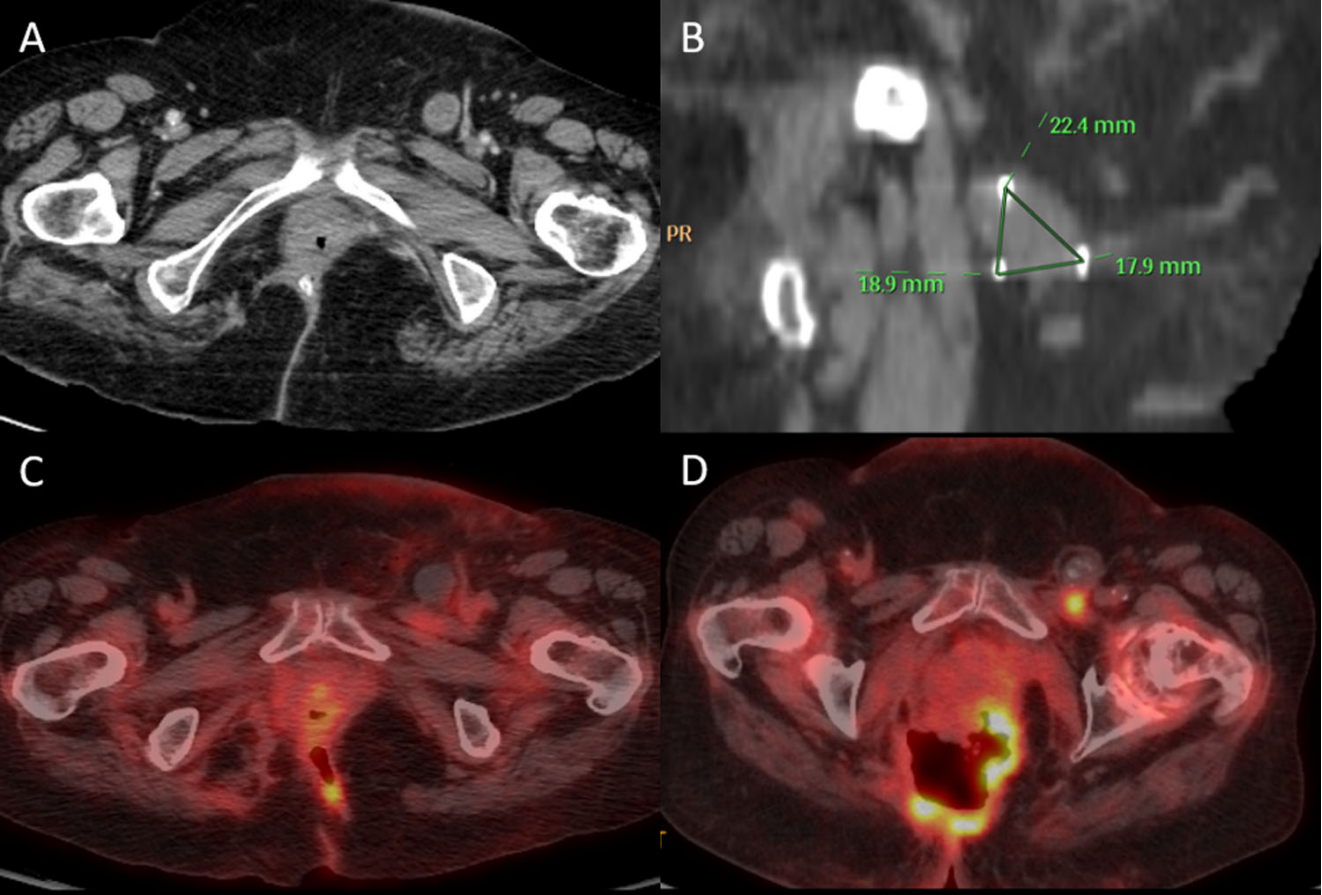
A 66-year-old female admitted with rectal pain. Past medical history of adenocarcinoma of the rectum diagnosed in 2019, underwent loop sigmoid colostomy, completed radiation and is receiving adjuvant chemotherapy (FOLFOX) upon treatment. (A) Abdominopelvic CT shows a left inguinal lymph node measuring up to 2.7 cm concerning for metastasis. Ultrasound-guided biopsy of the left inguinal lymph node with frozen samples was positive for adenocarcinoma. (B) Intraprocedural CT demonstrating intermittent placement of three 17-gauge NanoKnife IRE ablation needles. (C) Follow-up PET-CT the following day showing lack of FDG-uptake of the treated lymph node. (D) Follow-up PET-CT after 29 months showing FDG-uptake of the treated lymph node
